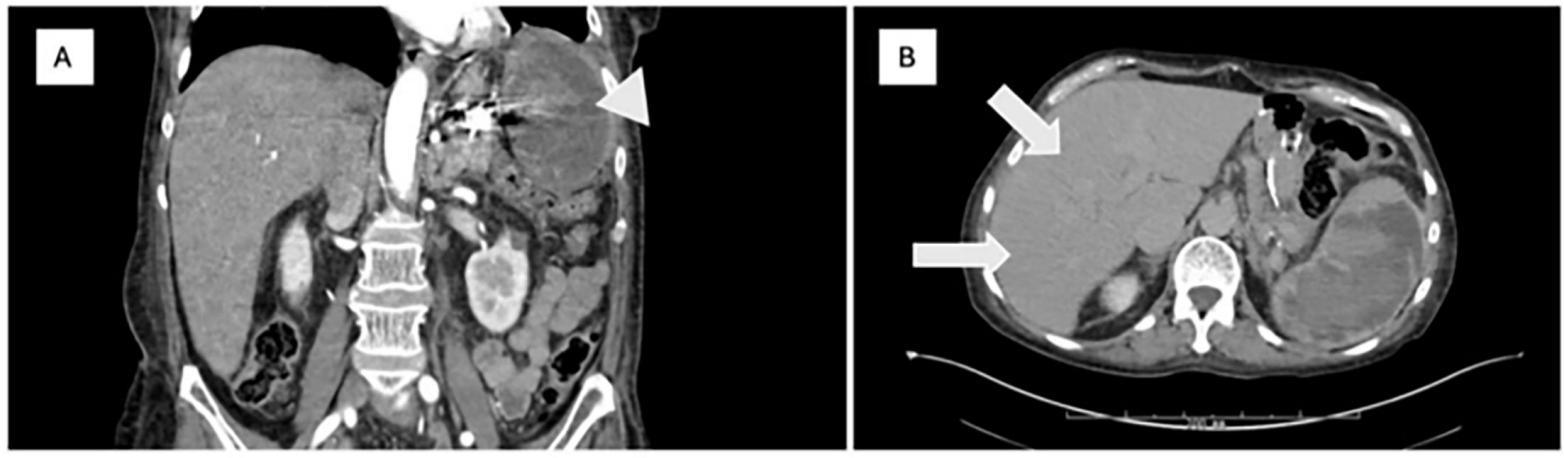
Coronal (A) and axial (B) computed tomographic imaging with contrast of the abdomen demonstrating splenic necrosis (arrowhead) and hepatic hypodensities (arrows), respectively.
